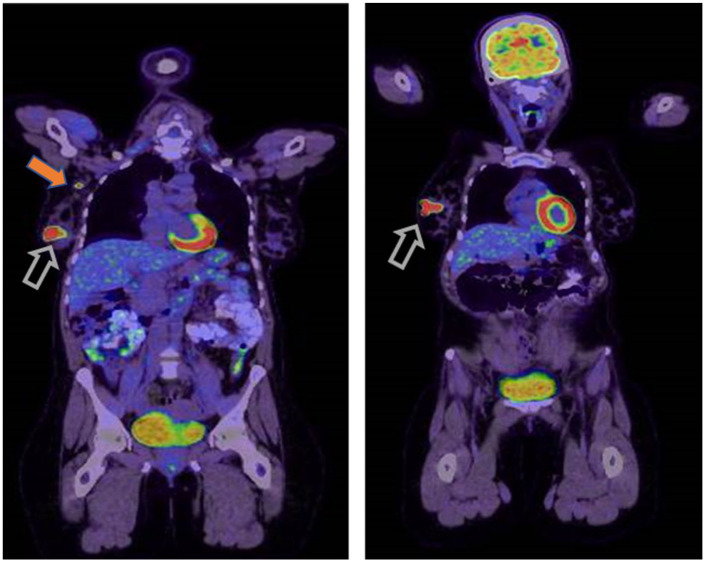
PET–CT scan showed two lobulated hypermetabolic masses in the lower quadrant of the right breast (open arrows) and an intensely hypermetabolic lymph node in the right axilla (block arrow). PET–CT: positron emission tomography–computed tomography.
